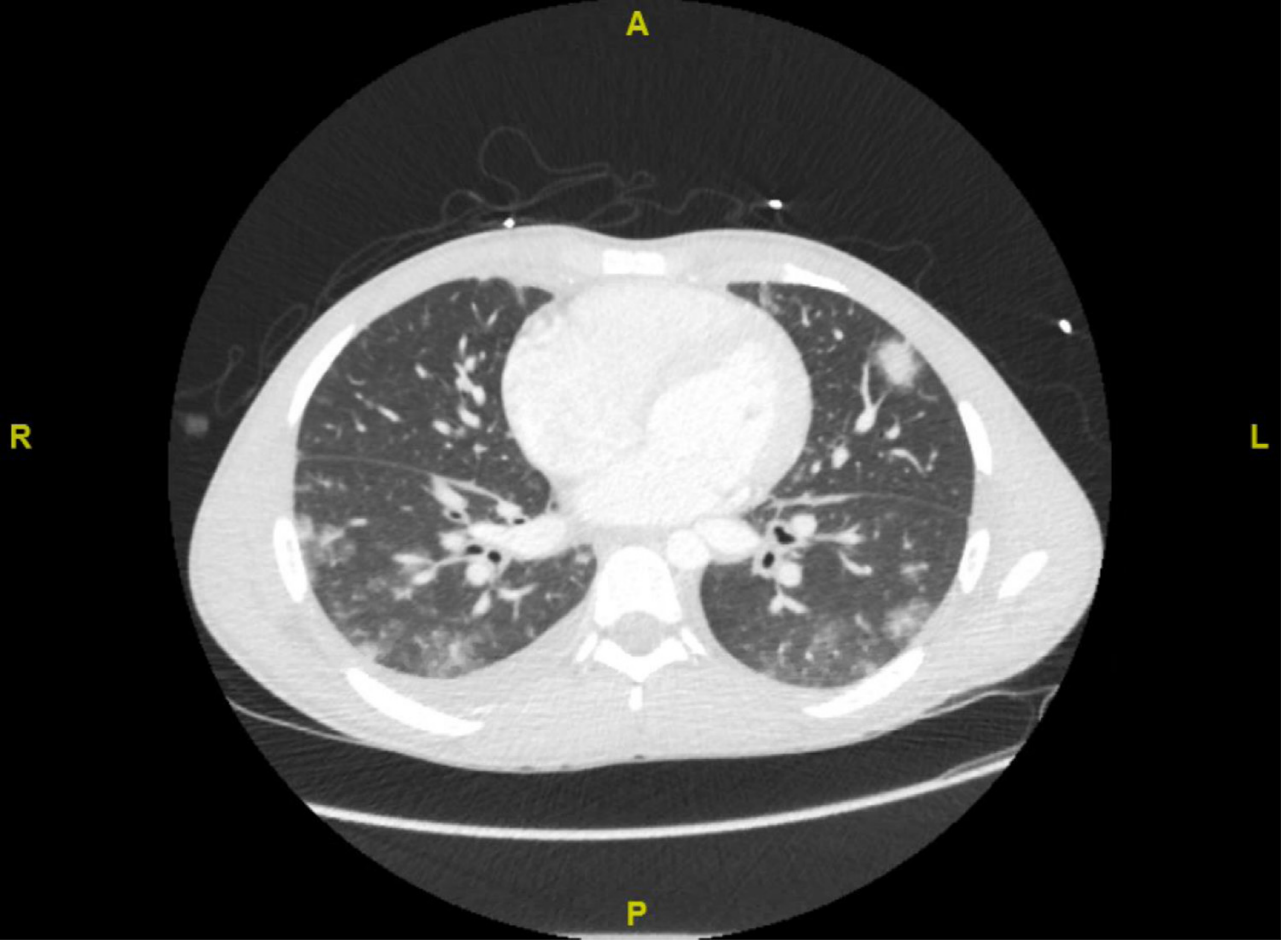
CT scan of the chest from Day 2 of the 1st hospitalization, showing diffuse ground-glass attenuation and consolidation of the lungs with lower lobe predominance.
